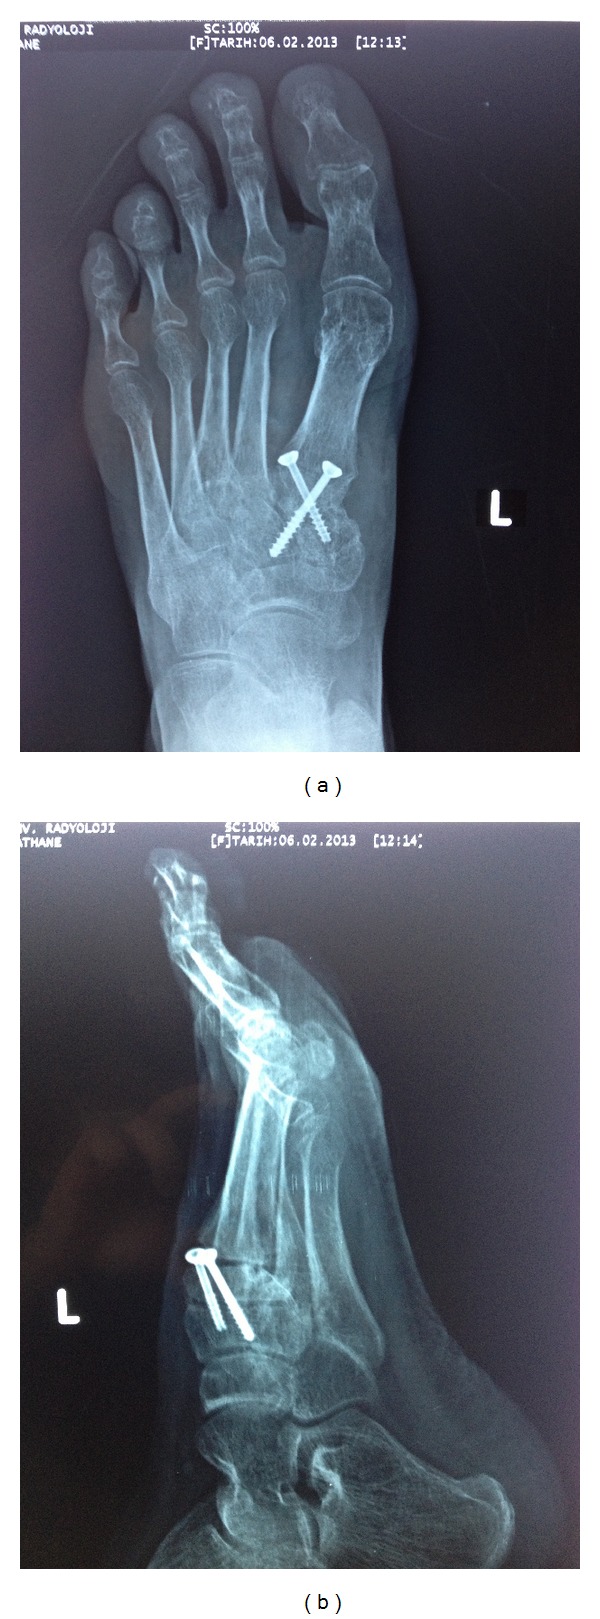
(a) Anteroposterior X-ray view 7 months after surgery. (b) Lateral X-ray view 7 months after surgery.
